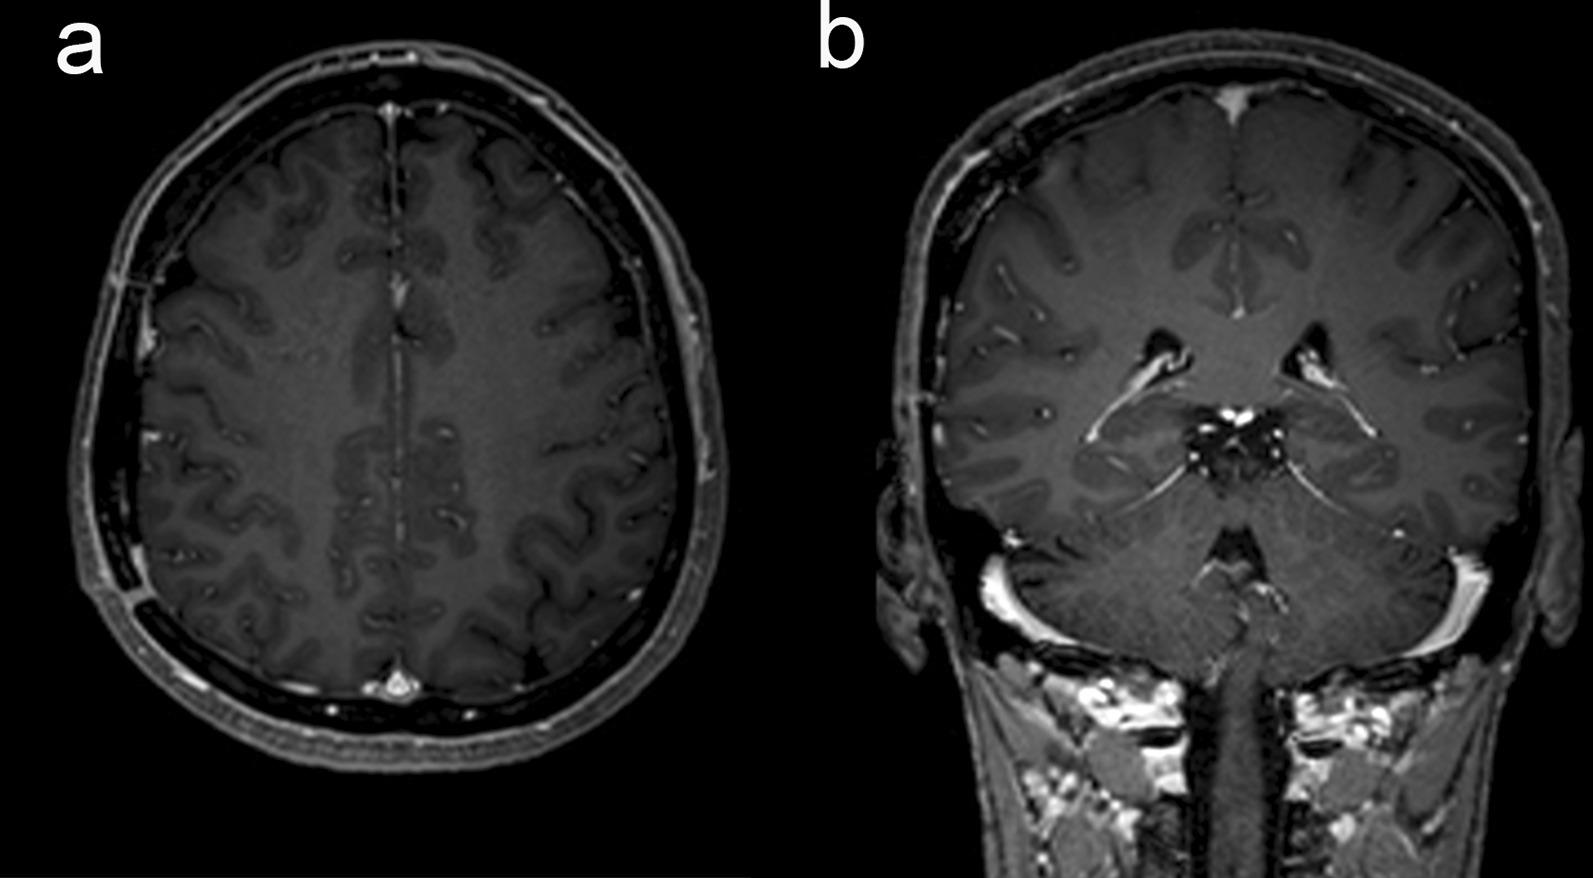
Six-month follow-up brain magnetic resonance images revealing no residual meningioma or subdural hematoma
